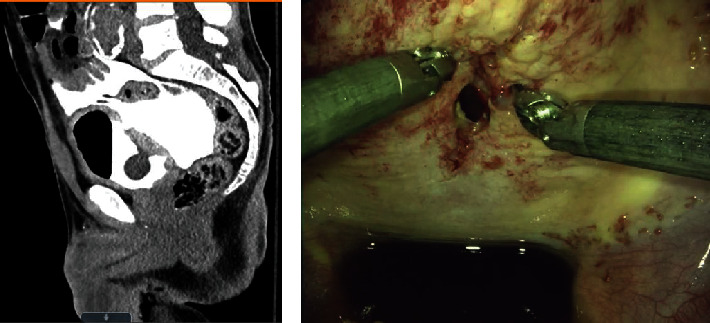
Intraperitoneal bladder injury. Computed tomography cystogram sagittal view demonstrated an intraperitoneal bladder injury at the dome. Direct robot-assisted laparoscopic visualization is shown. Repair was performed in a standard 2-layer fashion using 3-0 and 2-0 V-Loc™ sutures.
